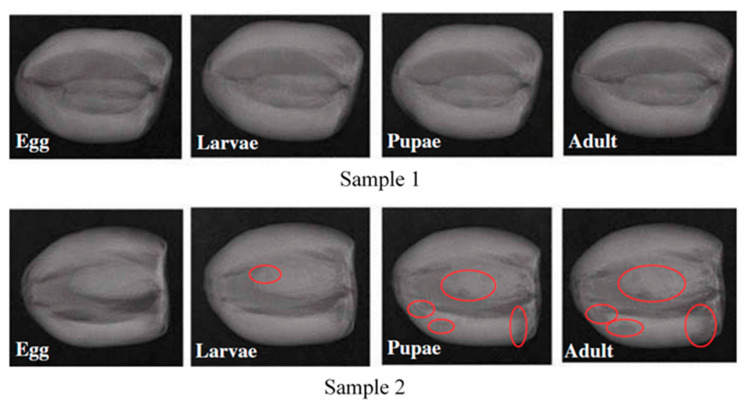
X-ray images of two samples of dates infested with *Oryzaephilus surinamensis* at different life stages. Samples 1 show infestation that is not visually recognizable, and samples 2 show identifiable infestation in areas circled in red [76].
